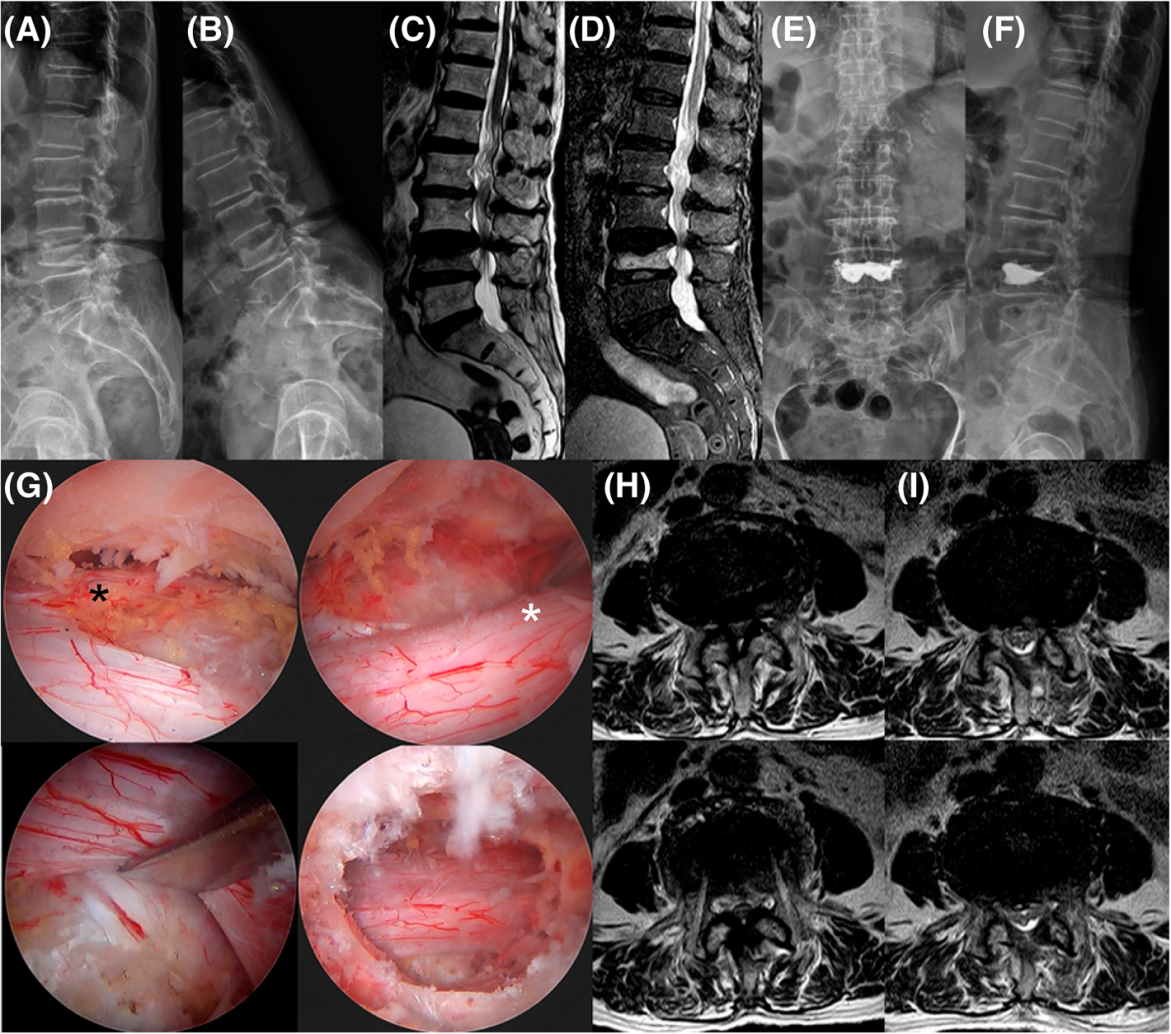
Representative clinical example where surgeries were performed using a uniform technique. A 90-year-old female patient was diagnosed with L4 osteoporotic vertebral collapse (concave-type), severe central canal and right foraminal stenosis at L3-L4, and L3 spondylolisthesis with instability. (A) Plain lateral radiographic image. (B) Dynamic flexion radiographic image. (C) Magnetic resonance T2-weighted sagittal image. (D) Magnetic resonance T1-weighted fat-suppression sagittal image. (E, F) The patient underwent percutaneous balloon kyphoplasty for L4 osteoporotic vertebral collapse but continued to experience severe radicular pain and its related gait disturbance. (G) Finally, she underwent unilateral biportal endoscopic decompressive laminectomy. Sufficient neural decompression of the right L3 exiting nerve root (black star), right L4 traversing nerve root (white star), and left L4 traversing nerve root (black cross) are shown by endoscopic visualization. The same can be seen on the preoperative (H) and postoperative (I) magnetic resonance T2-weighted axial images
